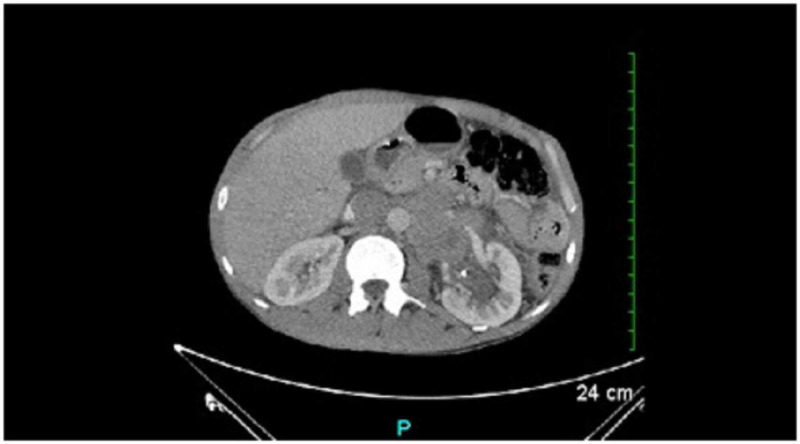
CT scan of the abdomen showing retroperitoneal mass measuring 4.7 x 6.3 x 7 cm and ureteral stent
